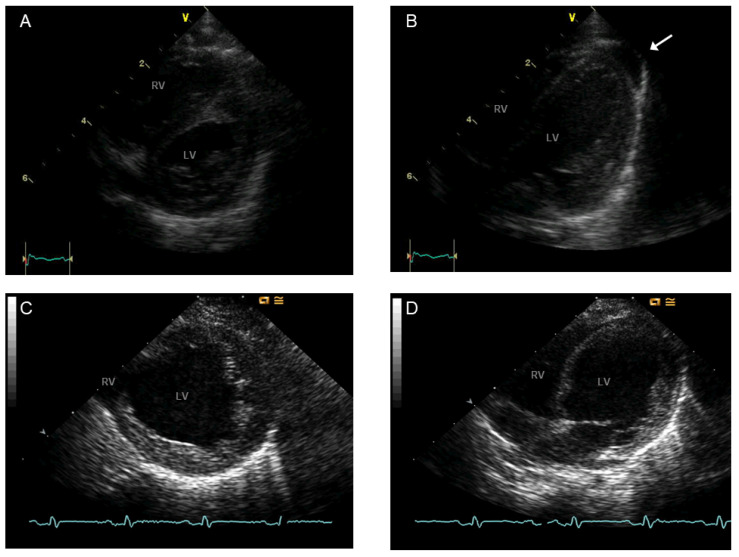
Echocardiography at the age of 2 weeks (A,B) and 8 months (C,D) of a patient with BTHS: Parasternal short axis view (left row) and atypical four-chamber view (right row). Normal systolic LV function (A,B) and minor pericardial effusion (arrow) at 2 weeks. Marked systolic dysfunction and LV dilatation at the age of 8 months, before heart transplantation (C,D). Abbreviations: RV, right ventricle; LV, left ventricle. Adapted from the following source: Karall et al. [22]. This is an open-access article published and distributed under the terms of the Creative Commons Attribution License, which permits unrestricted use, distribution, and reproduction in any medium, provided the original author and source are credited.
